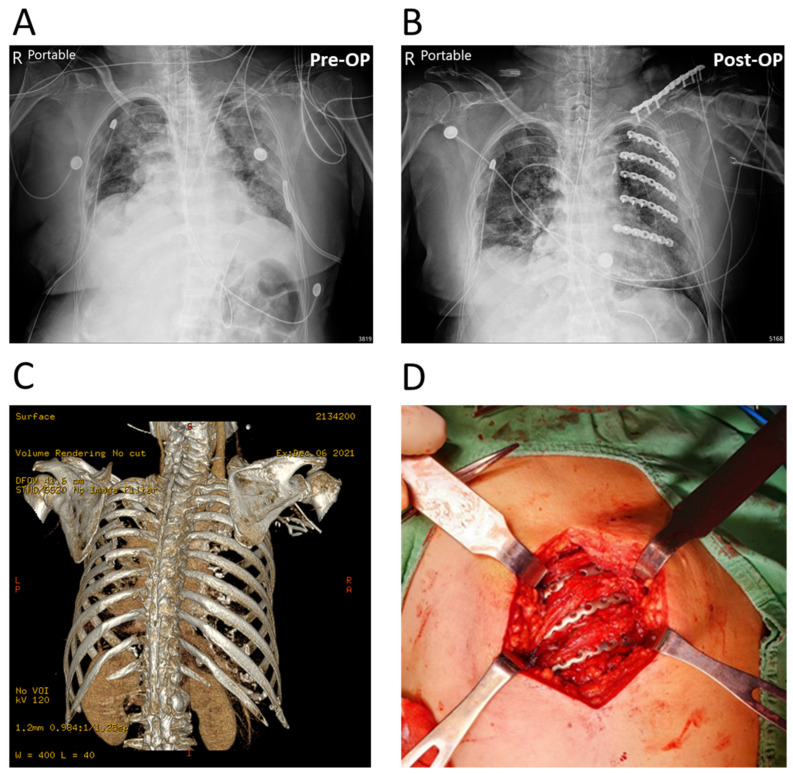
A representative case of multiple rib fractures repaired with APS plates. (A,B) AP radiograph of pre-operation (Pre-OP) and post-operation (Post-OP). (C) Three-dimensional CT reconstruction image of the injury. (D) Intraoperative photograph showing the rib-repairing technique with implanted APS plates.
